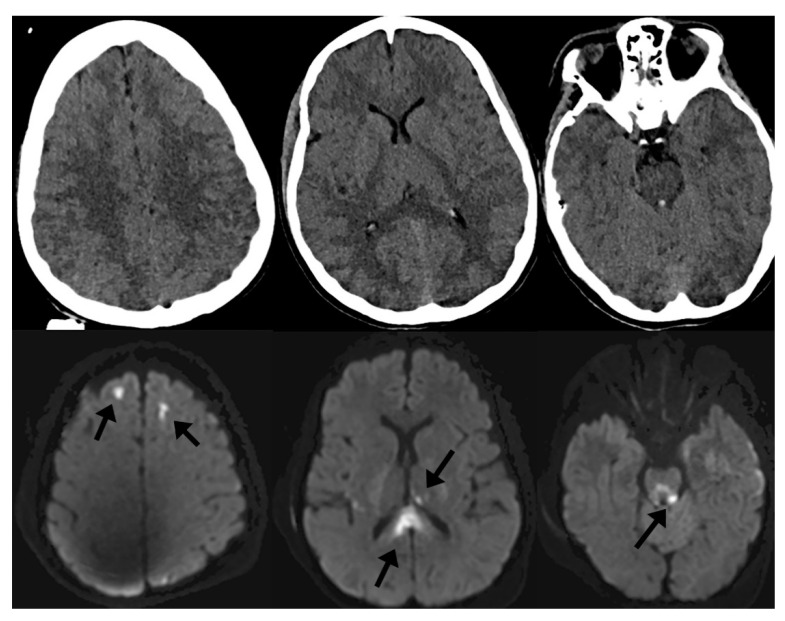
Noncontrast computed tomography (CT) of the head (top row) and diffusion-weighted imaging (DWI) of the brain (bottom row) of the same patient after traumatic brain injury (TBI). Imaging markers of diffuse axonal injury (DAI) include microhemorrhages on susceptibility-weighted imaging (SWI) as well as focal areas of cytotoxic edema on DWI (arrows) that are not visible on CT.
